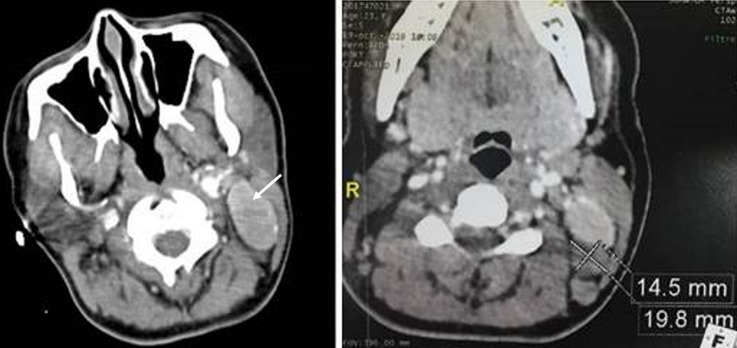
cervical CT scan with contrast enhancement showing cervical lymph node in left IIB and V levels with a strong enhancement of the IIB level lymph node (white arrow)
